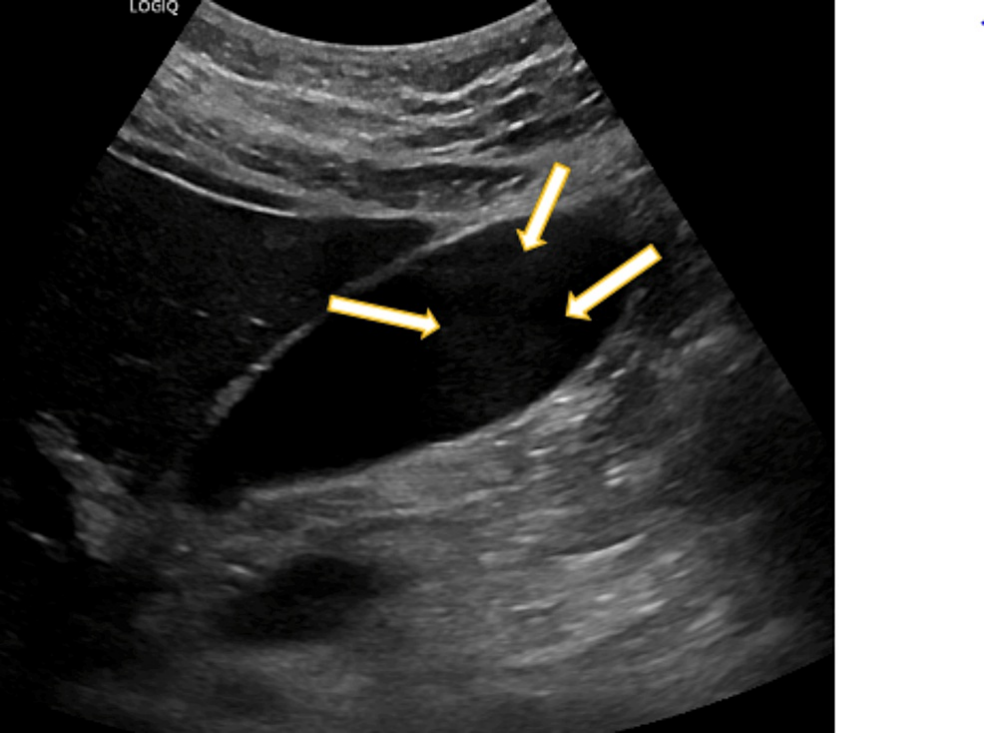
Ultrasound of liver. Sludge is present within the gallbladder fossa (yellow arrows). Views provided of the gallbladder demonstrate some low-level echoes within the gallbladder fossa with a fluid/debris level compatible with sludge.
